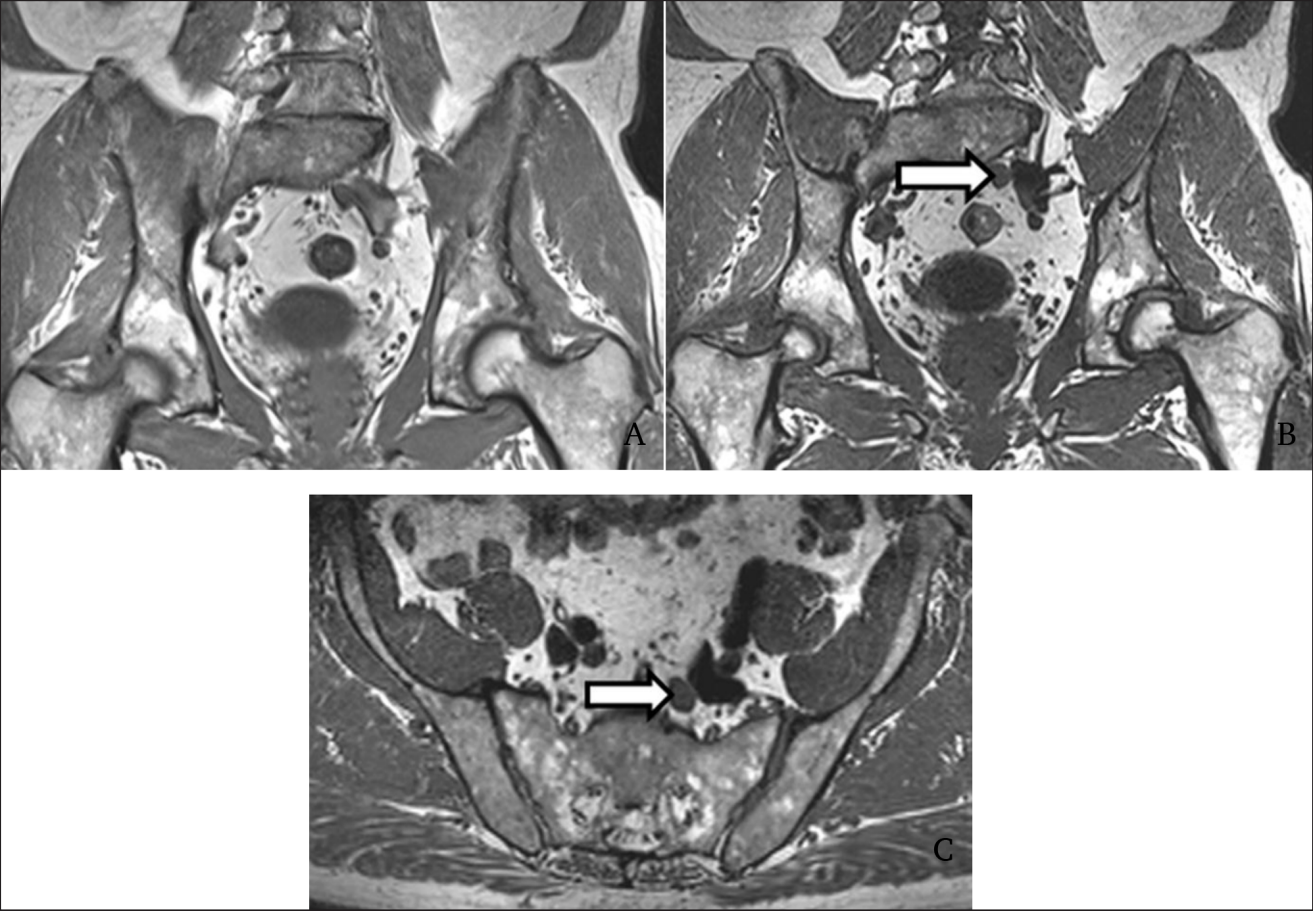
Detection of a node in a 57-year-old PCa patient. The enlarged LN was missed by both readers on 2DT1 sequence (A) but correctly identified by both on 3D T1 sequence (B). Axial reconstruction obtained with multiplanar reformation at same level confirms the presence of an enlarged LN (C). Reprinted from ‘Whole-body 3D T1-weighted MR imaging in patients with prostate cancer: feasibility and evaluation in screening for metastatic disease’ Pasoglou V., Michoux N., Peeters F., Larbi A., Tombal B. Omoumi P., Vande Berg B., Lecouvet F.E, Radiology. 2015 Apr; 275(1): 155–66. [43] With the permission of RSNA.
